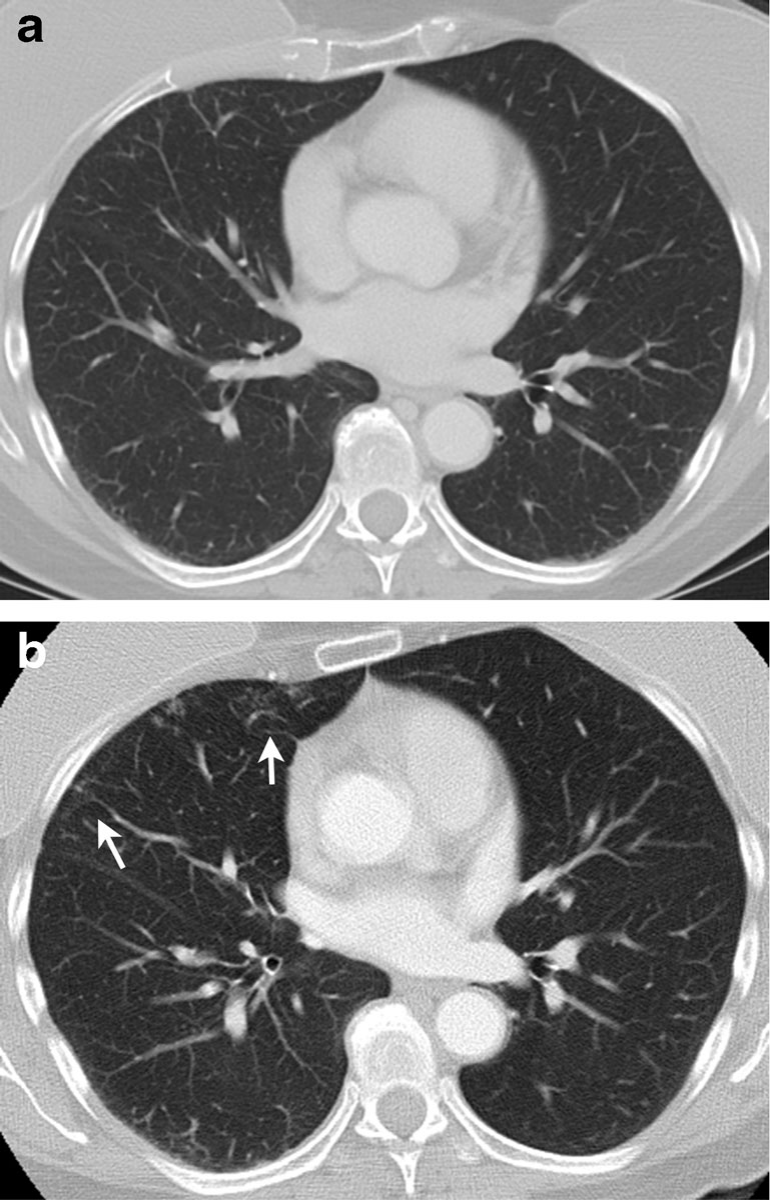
67-year-old woman with metastatic HER2/neu-positive breast cancer being treated with trastuzumab. Patient had prior radiotherapy in right breast. Axial contrast-enhanced lung window CT image before (a) and after (b) treatment with trastuzumab shows interval appearance of peripheral ground-glass opacities in right middle lobe (*arrows* in b) in radiation field suggestive of radiation recall pneumonitis
